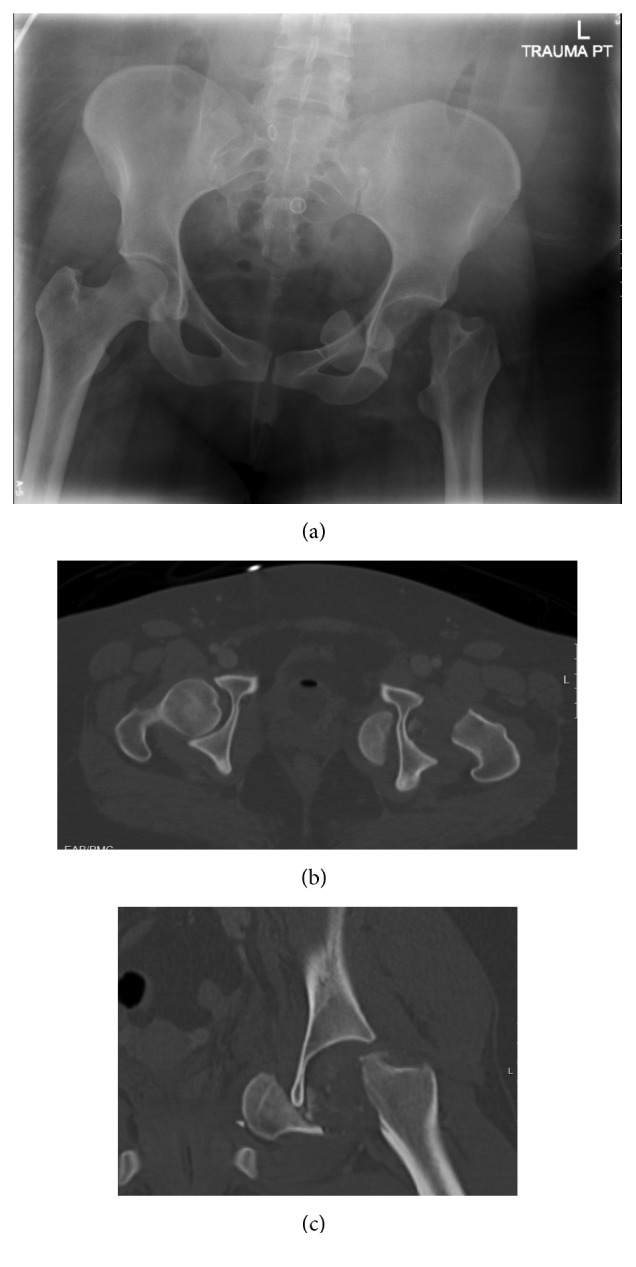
(a) Anteroposterior radiograph demonstrating complete medially displaced left femoral neck fracture. (b, c) Computed tomography (CT) demonstrating the displacement of femoral head in the intrapelvic location, which had extruded through the obturator foramen.
